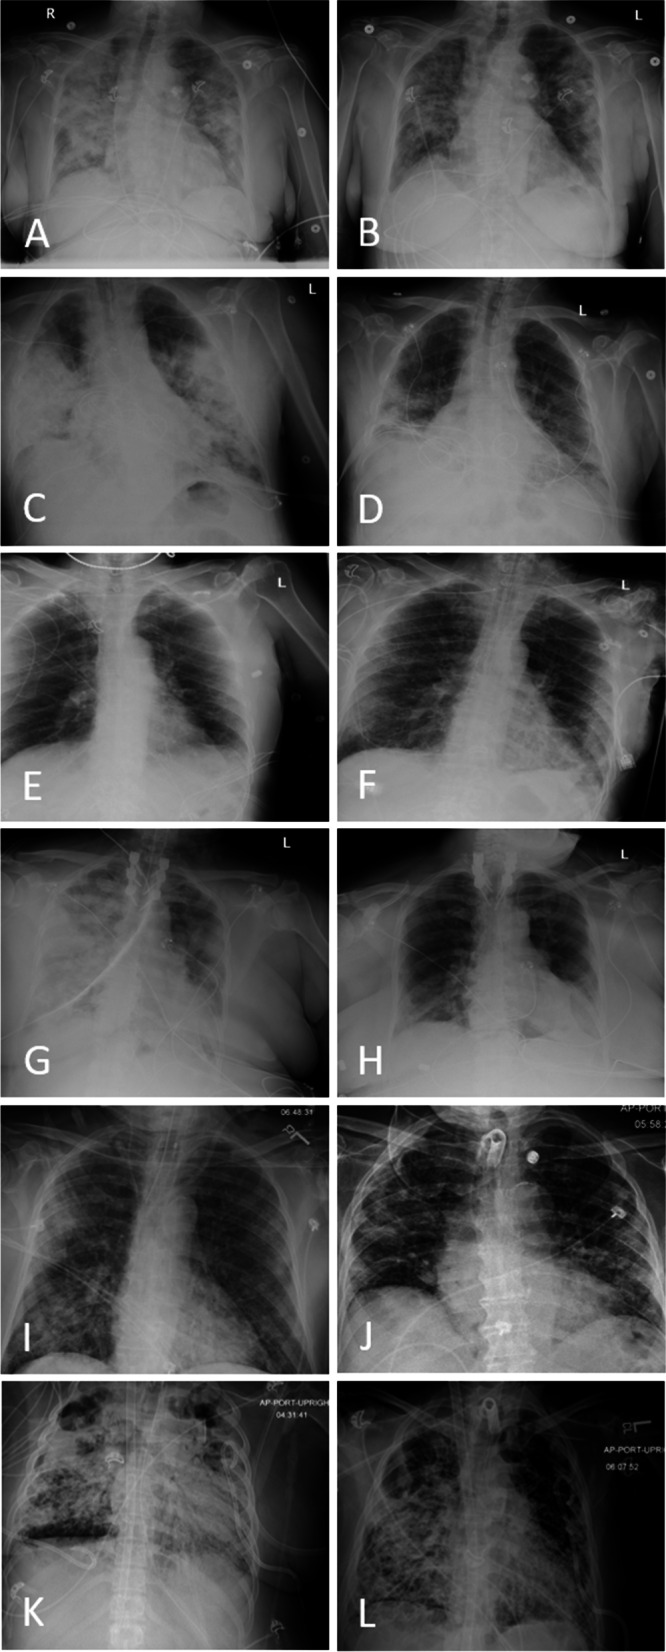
Chest radiographs of patient prior to and following treatment with Mesenchymal Stem Cells (MSCs). Seventy-nine year old female, with MSC treatment 2 days post-admission; chest radiograph day 0 showing bilateral infiltrates (A) and 12 days post-treatment (B). Sixty-four year old critically severe male, radiograph on day of ventilation and MSC treatment (C). Marked improvement of infiltrates and extubation 8 days following initial MSC infusion (D). 73 year old critically severe male treated with MSCs 12 days after initial admission. Radiograph the day prior to MSC treatment (E) indicates pulmonary infiltrates, which improved by day 9 following MSC infusion (F). Sixty-one year old female categorized as critically severe COVID-19 on admission, treated with MSCs 4 days following admission. Chest x-rays one day prior to MSC infusions indicates severe pulmonary infection (G) which substantially improved by day 11 post-treatment (H). Eighty-four year old male treated with MSCs 11 days after admission. Pre-treatment radiograph (I) on day 11 shows mild worsening of patchy airspace opacities bilaterally. There was slow improvement over time and infiltrates reduced on day 22 following MSC treatment (J). 30 year old female treated with MSCs 57 days after admission. Pre-treatment radiograph (K) on day 56 shows stable severe diffuse multifocal pulmonary opacities and focal lucencies within both upper lung zones which may represent loculated pleural air or parenchymal cavitation. Infiltrates reduced on day 4 following MSC treatment (L)
